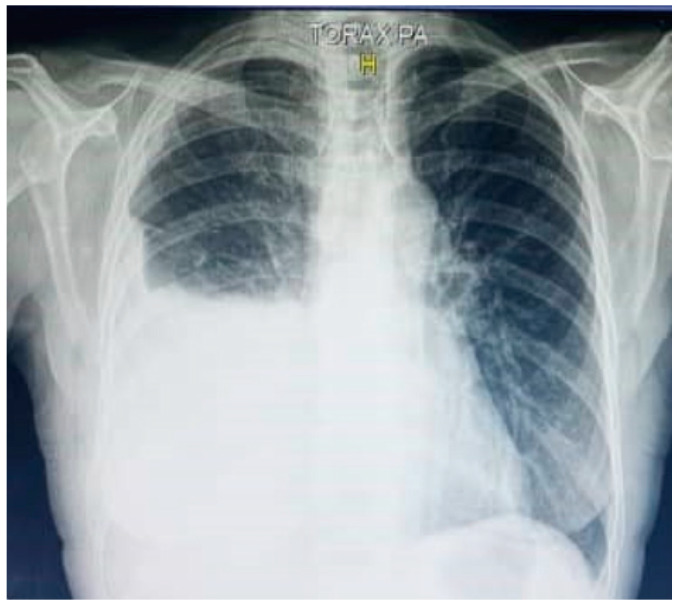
Posteroanterior chest X-ray with a typical lung collapse image of pleural effusion. A dense, homogeneous opacity at the lower two-thirds of the right hemithorax obscures the ipsilateral cardiac and diaphragmatic borders.
